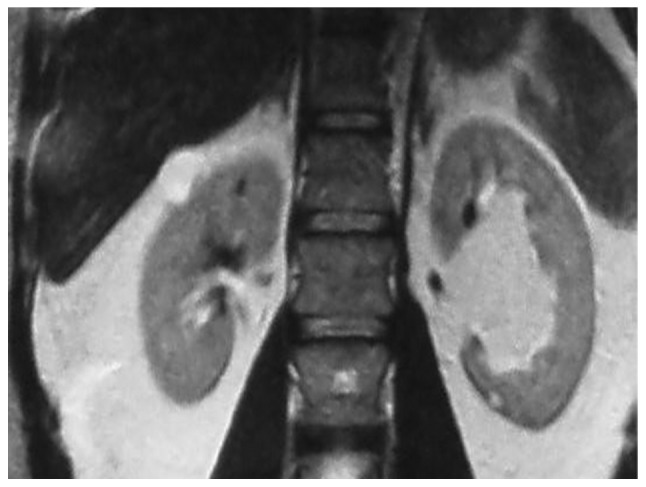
Case 3. Coronal magnetic resonance imaging examination of the kidney revealed an 8×5×5 cm lesion situated in the left renal sinus. Results showed a high signal on T1 weighted image (WI) and T2WI, but a low signal in fat suppression imaging.
